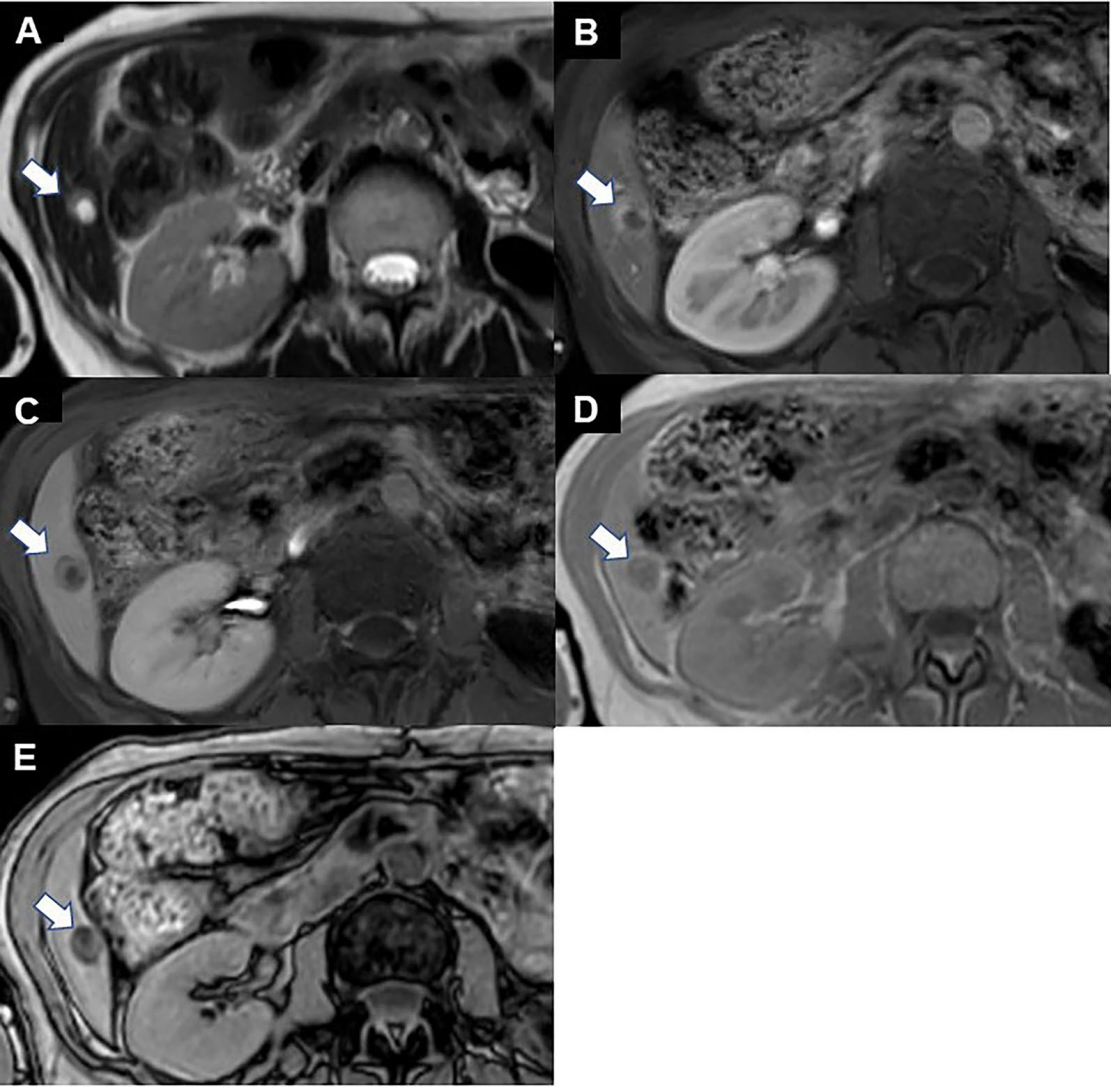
Dynamic contrast-enhanced MRI of the abdomen. A A 16-mm mass lesion with both cystic and solid, lipid-containing components (arrow, T2WI). B Arterial phase (T1W1). C Portal phase (T1W1). The solid components demonstrated arterial enhancement followed by rapid washout in the portal phase (arrow, T1WI). D In-phase (T1W1). E Opposed-phase (T1W1). A signal drop can be observed on the opposed-phase image compared to the in-phase image (arrow)
